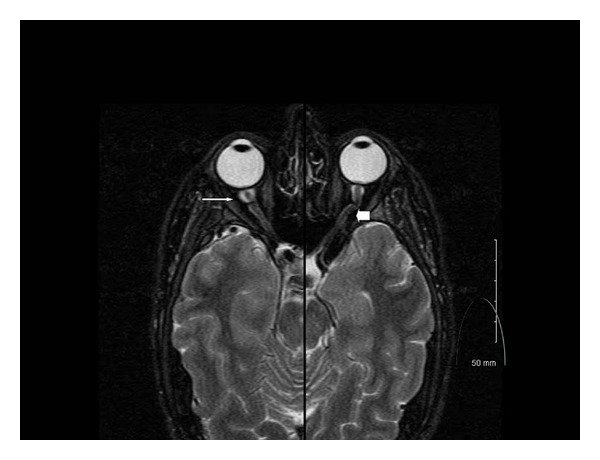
MRI of optic nerves. Merged axial T2 MRI at the level of the optic nerves shows significantly increased optic nerve sheath expansion near the globe (thin arrow), which extends through the course of the optic nerve sheath (thick arrow).
